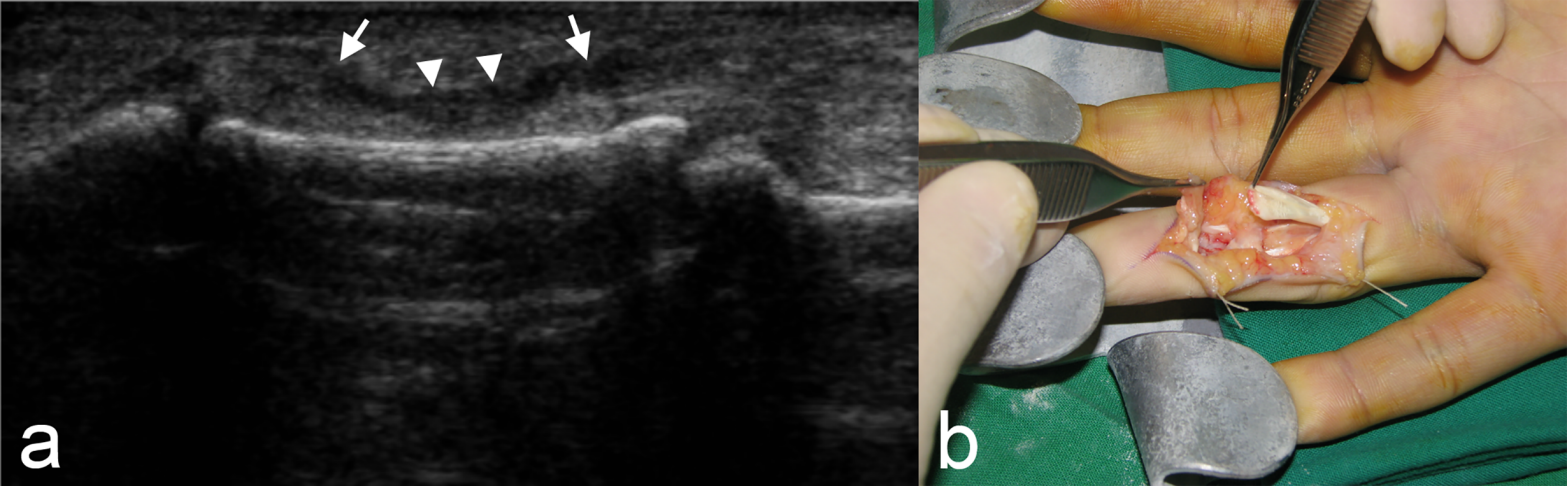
A 23-year-old man with a flexor digitorum profundus tendon rupture of the ring finger. (a) Ultrasound image of longitudinal scan shows discontinuity of the tendon with retraction of the ruptured tendon (arrows) at the middle phalangeal level. Decreased echogenicity of the ruptured tendon site is also seen (arrowheads). (b) On surgery, the rupture of the flexor digitorum profundus tendon was confirmed and picked up with forceps.
